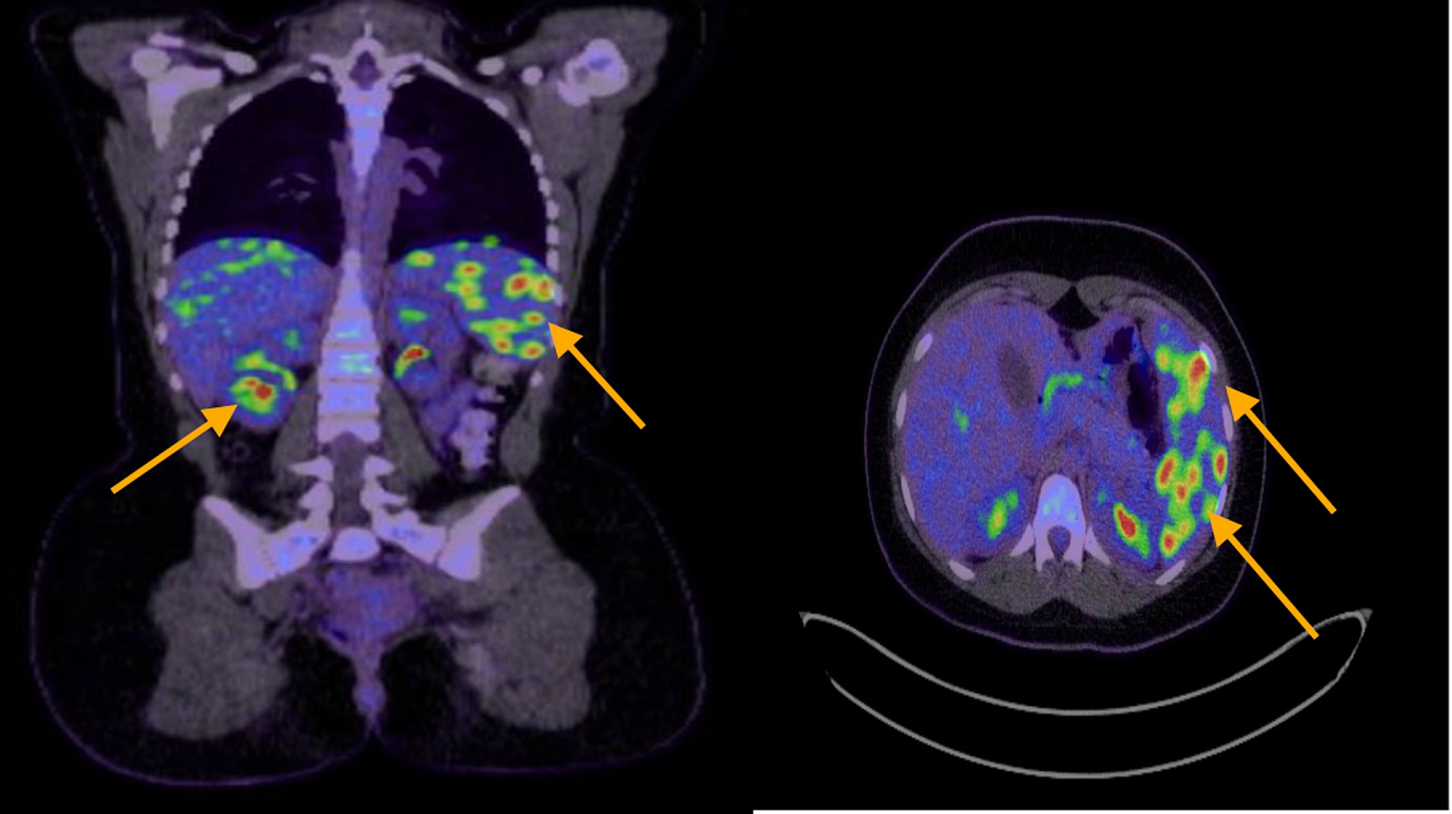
PET FDG scan showing increased multiple focal uptakes in the liver and spleen (marked by yellow arrows) PET, positron emission tomography; FDG, fluorodeoxyglucose
